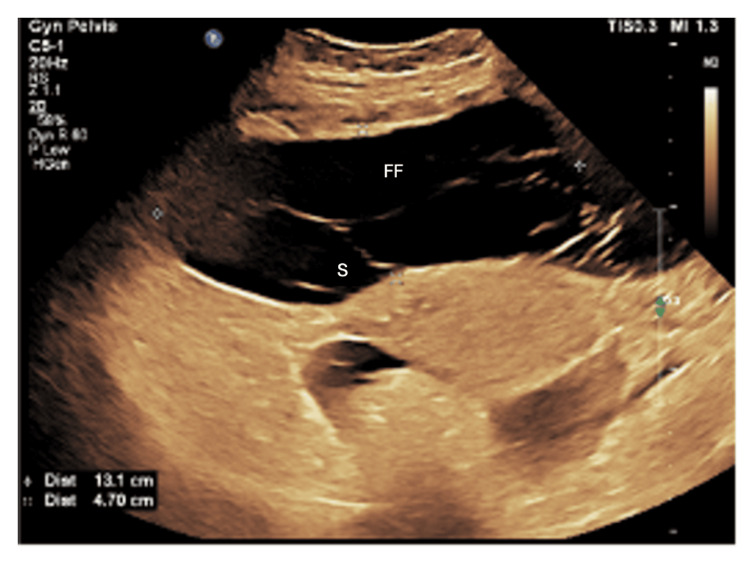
Free fluid with multiple internal sepatations inside detected by ultrasonopgraphy This is an original image. FF: Free fluid, S: Separation
